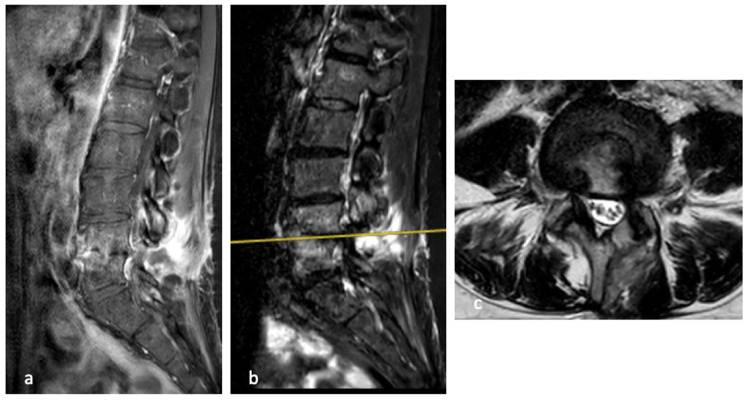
MRI at the resolution of the clinical symptoms and the improvement of the laboratory tests, alterations of the vertebral bodies are still visible in the sagittal T1 (a) and T2 (b) weighted sequences. In the axial view (c), the disc and root are less involved.
